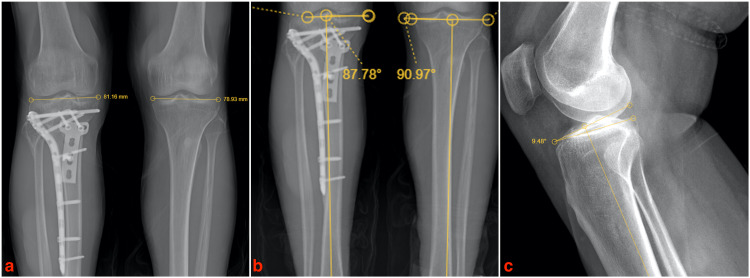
Measurement of the radiographic parameters used to evaluate the patients. (a) Measurement of the condylar width in both knees and the difference between them, (b) measurement of the medial proximal tibial angle in orthoroentgenogram and the difference between them and (c) measurement of the posterior tibial slope angle
